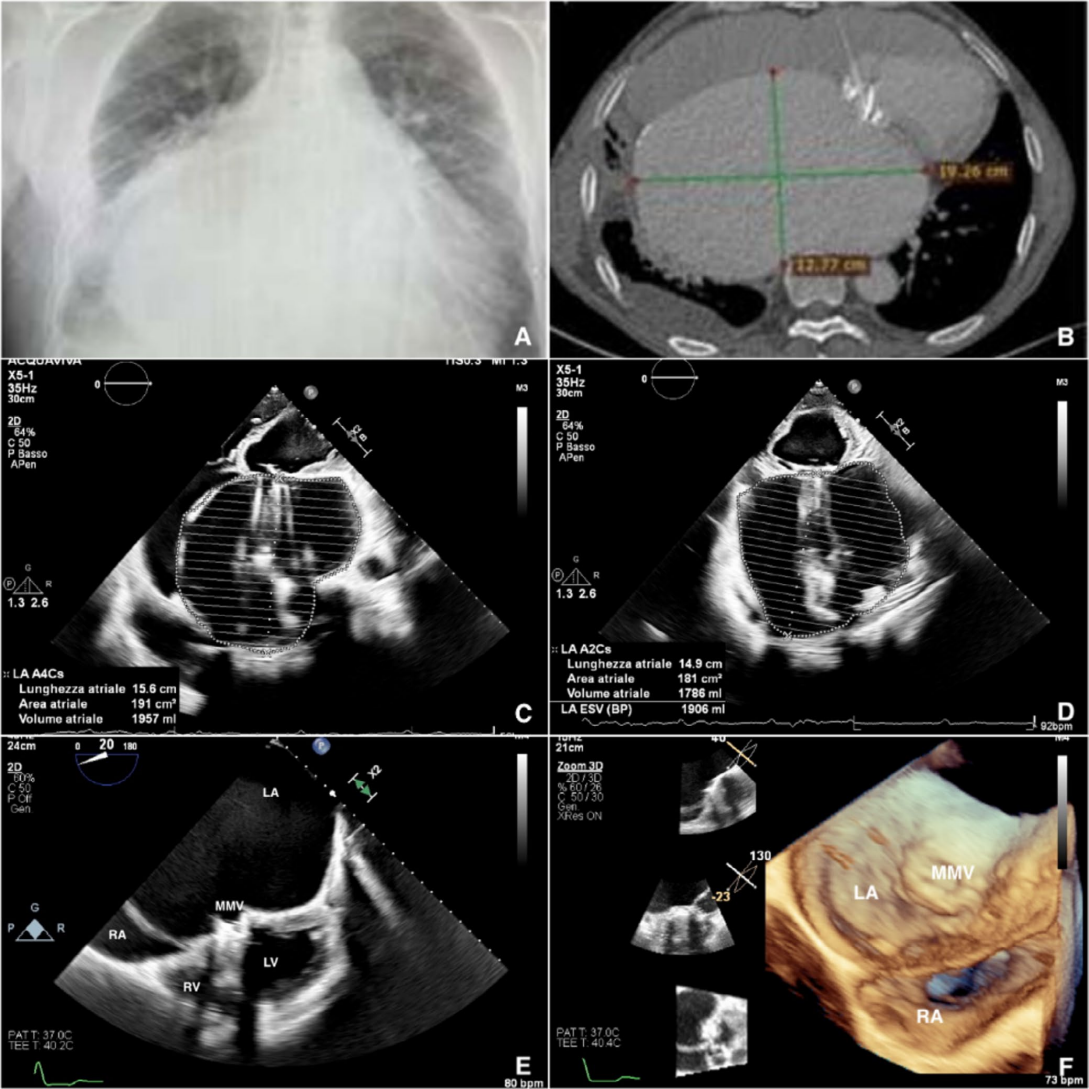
Pre‐operative imaging: Chest X ray (A); CT scan in coronal view (B); Transthoracic echocardiogram with end‐systolic LA biplane volume (C, D); Transesophageal echocardiogram 2D mid esophageal 4‐chamber view (E) and 3D real time view (F). LA, left atrium; LV, left ventricle; MMV, mechanical mitral valve; RA, right atrium; RV, right ventricle.
